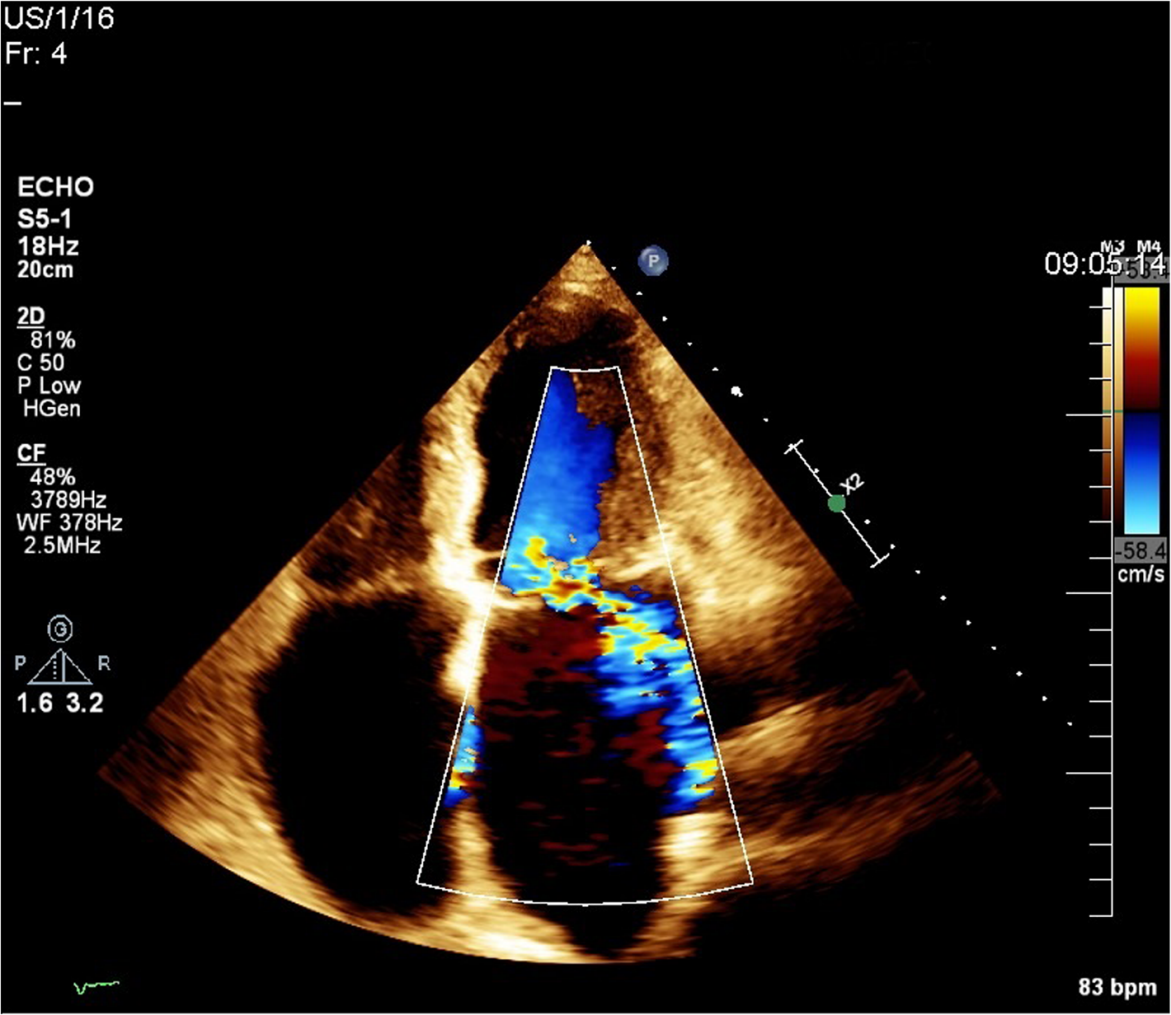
Echocardiogram on admission A four-chamber view showing giant LA, mitral annular dilatation with ruptured and flailed chordae tendinea of the anterior leaflet and significant mitral regurgitation
